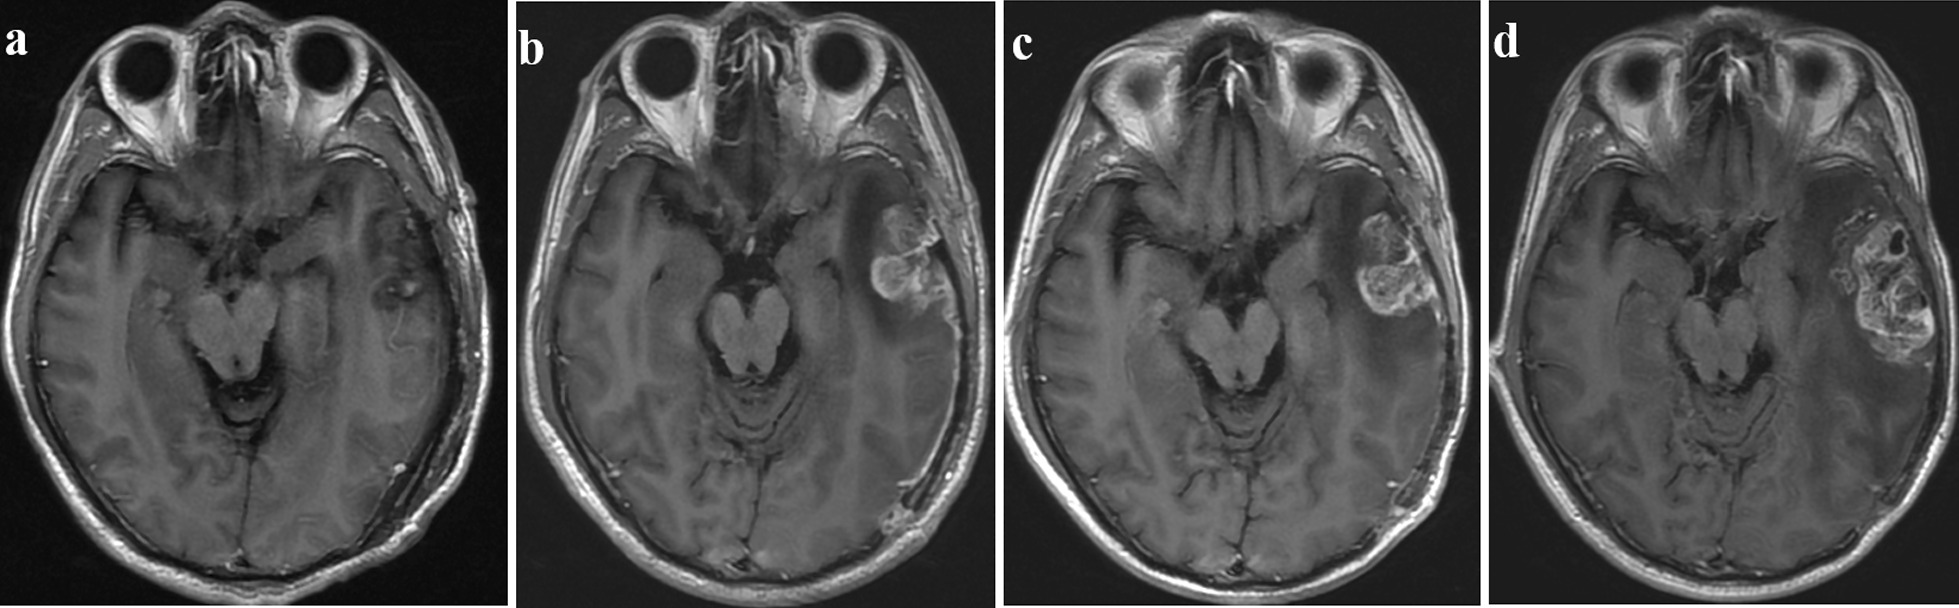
T_1_CE images showing true progression in a 48-year-old male patient with GBM. (a) Postoperative MRI (within 24 h after surgery) showed that the tumor was completely resected. (b) Two months after CCRT, the new enhancement disappeared. After CCRT and at the (c) 6-and (d) 9-month follow-ups, the follow-up MR images demonstrated that the extension of the enhanced lesion increased. Recurrence was confirmed by second surgical pathology. (GBM: glioblastoma multiforme)
